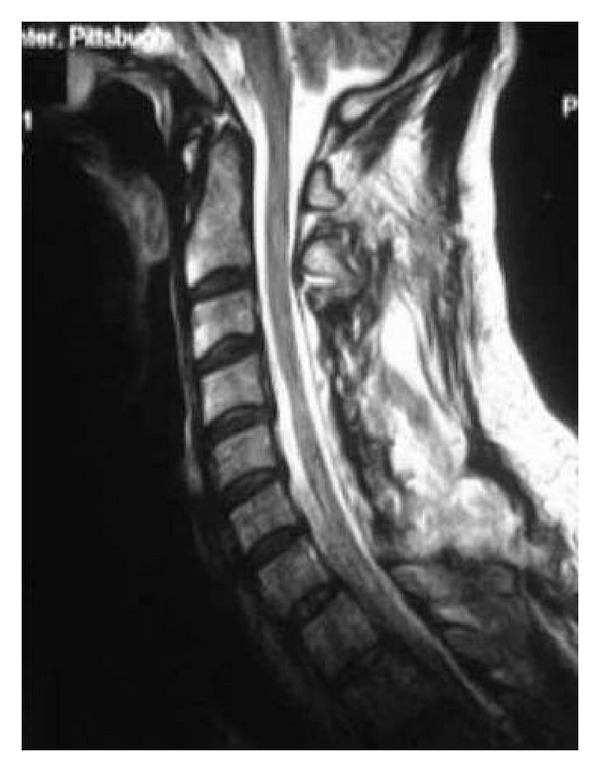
Post-laminoplasty MRI showing the space available for the cord created by the posterior decompression.
